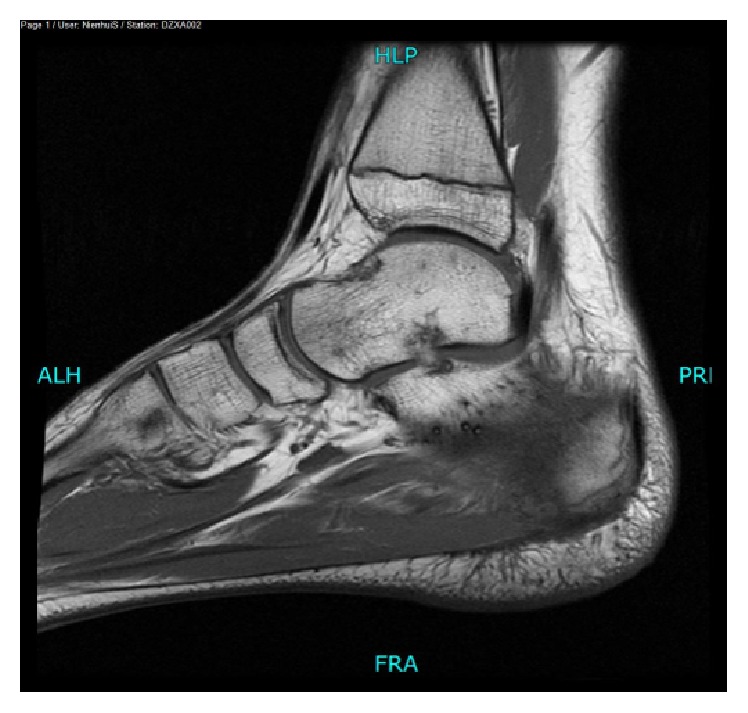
MRI T1: sagittal view of the left foot: enthesophyte at the insertion of the plantar fascia.
